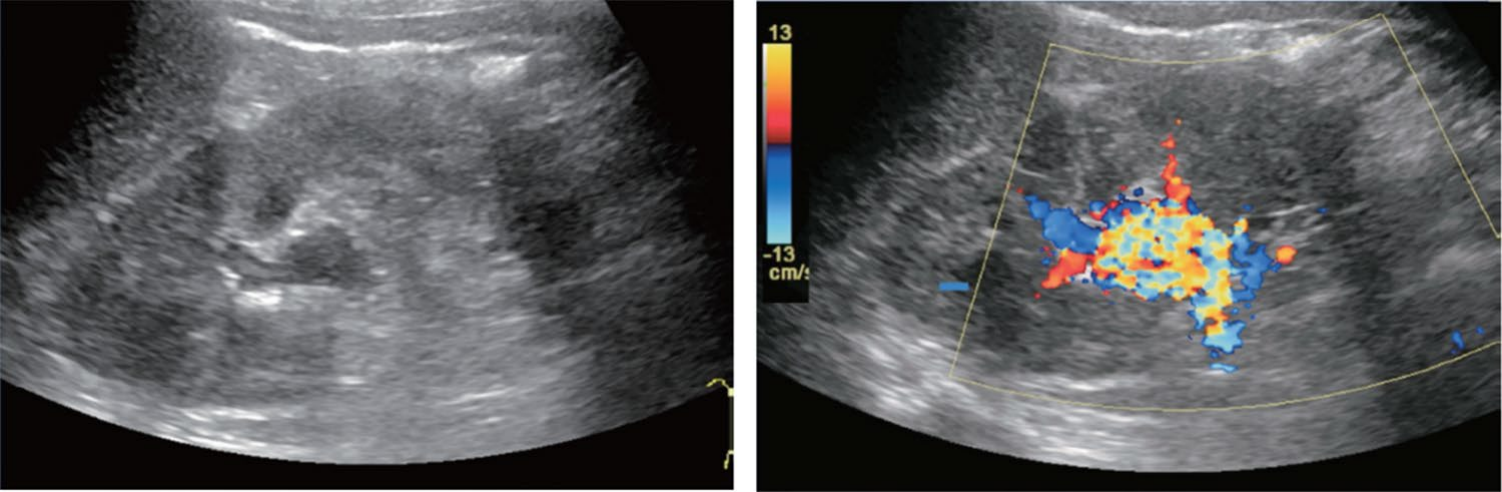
Other findings. Vascular abnormality. An irregular anechoic lesion is present in the renal central echo complex (left), and a blood flow signal is present on color Doppler (right). Left: renal long-axis view b-mode; right: color Doppler. Category 2, Assessment D2
